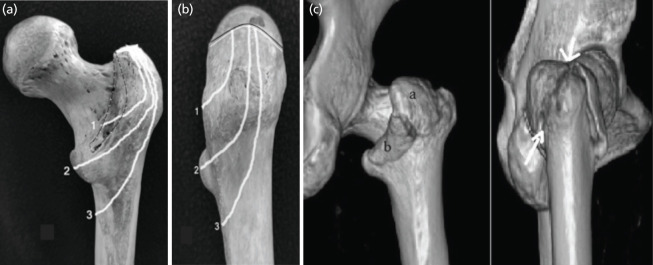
(a and b) Coronal fragment geometry where fracture line shows with white lines originating from trochanteric summit (black and white line in fig. b) exiting through ITC (1), LT (2) and PMC (3). (c) 3D CT scan NAKANO classification: type 1 3A fracture Coronal fragments: GT [a] LT [b] where its exit noted at ITC and LT (white arrow).
